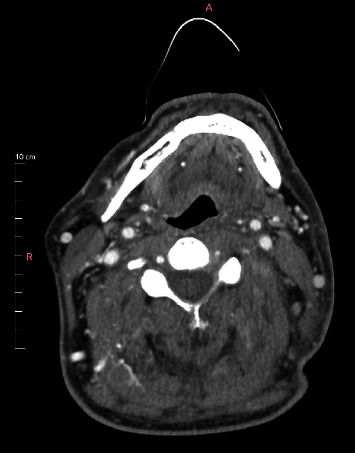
Contrast-enhanced neck CT showing persistence of the IJV thrombi, asymmetry of the left pharyngeal wall, and enlargement of the left carotid and post-styloid parapharyngeal spaces.
